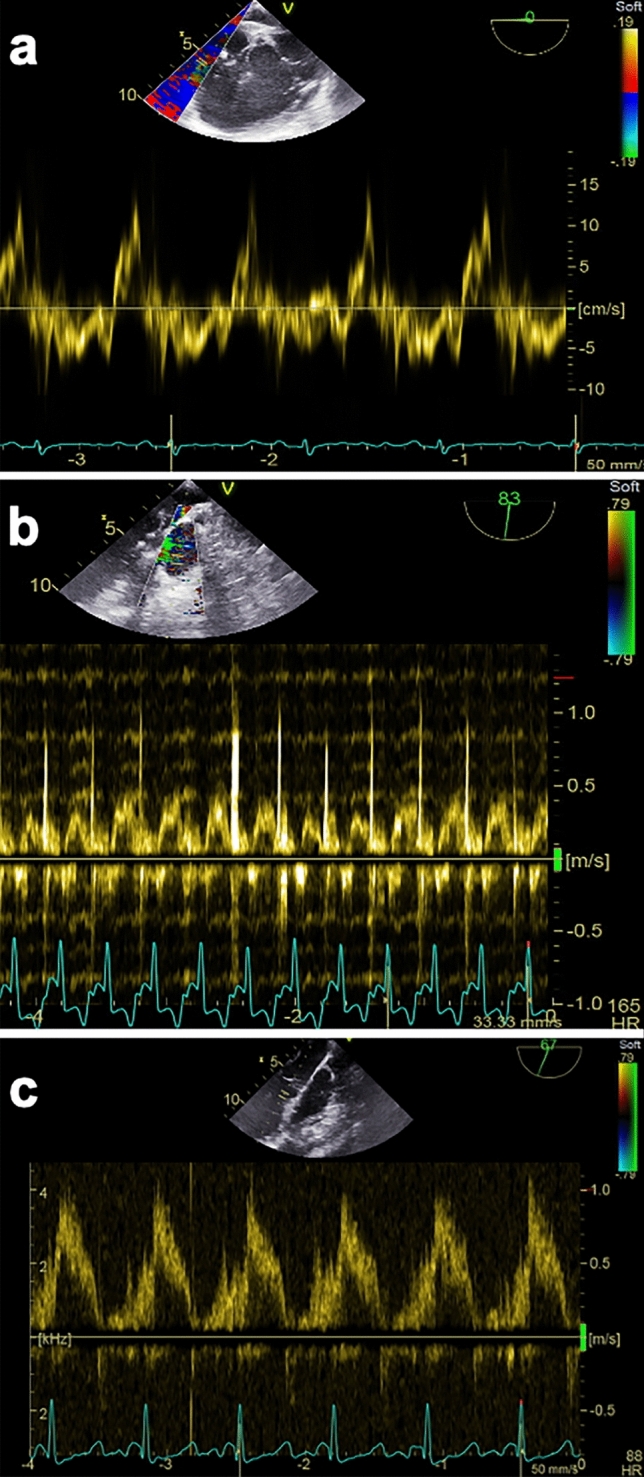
Representative TEE pulsed wave Doppler and tissue Doppler measurements. a Tissue Doppler beam aligned with the lateral tricuspidal annulus to measure tricuspidal annular plane systolic velocity (TASV). b TEE pulsed wave Doppler measurement in the right ventricular outflow tract. c TEE pulsed wave Doppler measurement in the left ventricular outflow tract
